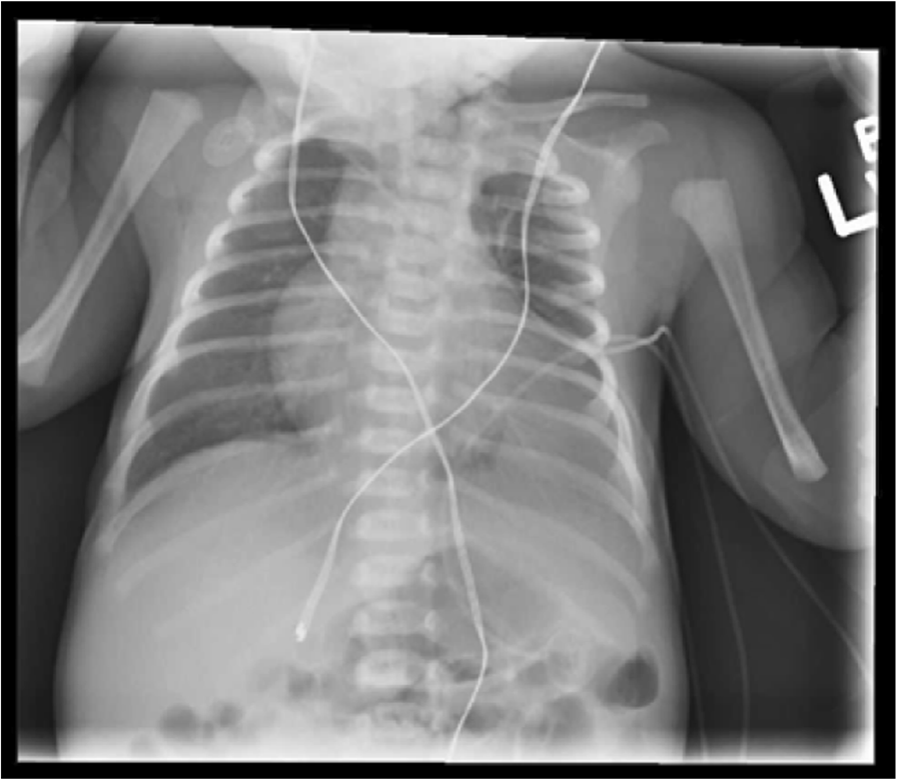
Pre-operative Chest X-Ray. Initial presenting chest X-ray demonstrating left lower lobe opacification
